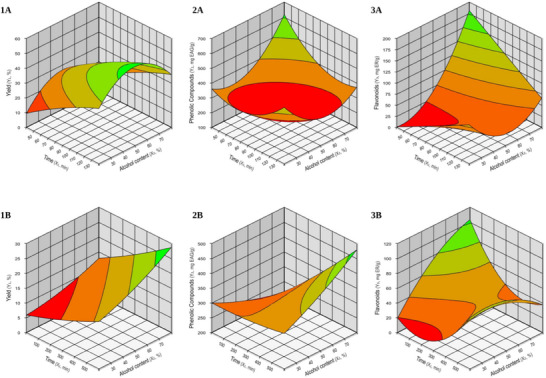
Response surface plots showing the effect of extraction time and ethanol concentration on: (1) Yield, (2) phenolic compound content, and (3) flavonoid content in extracts from *Cariniana rubra* bark obtained by (A) ultrasonic‐assisted extraction (UAE) and (B) Soxhlet apparatus.
